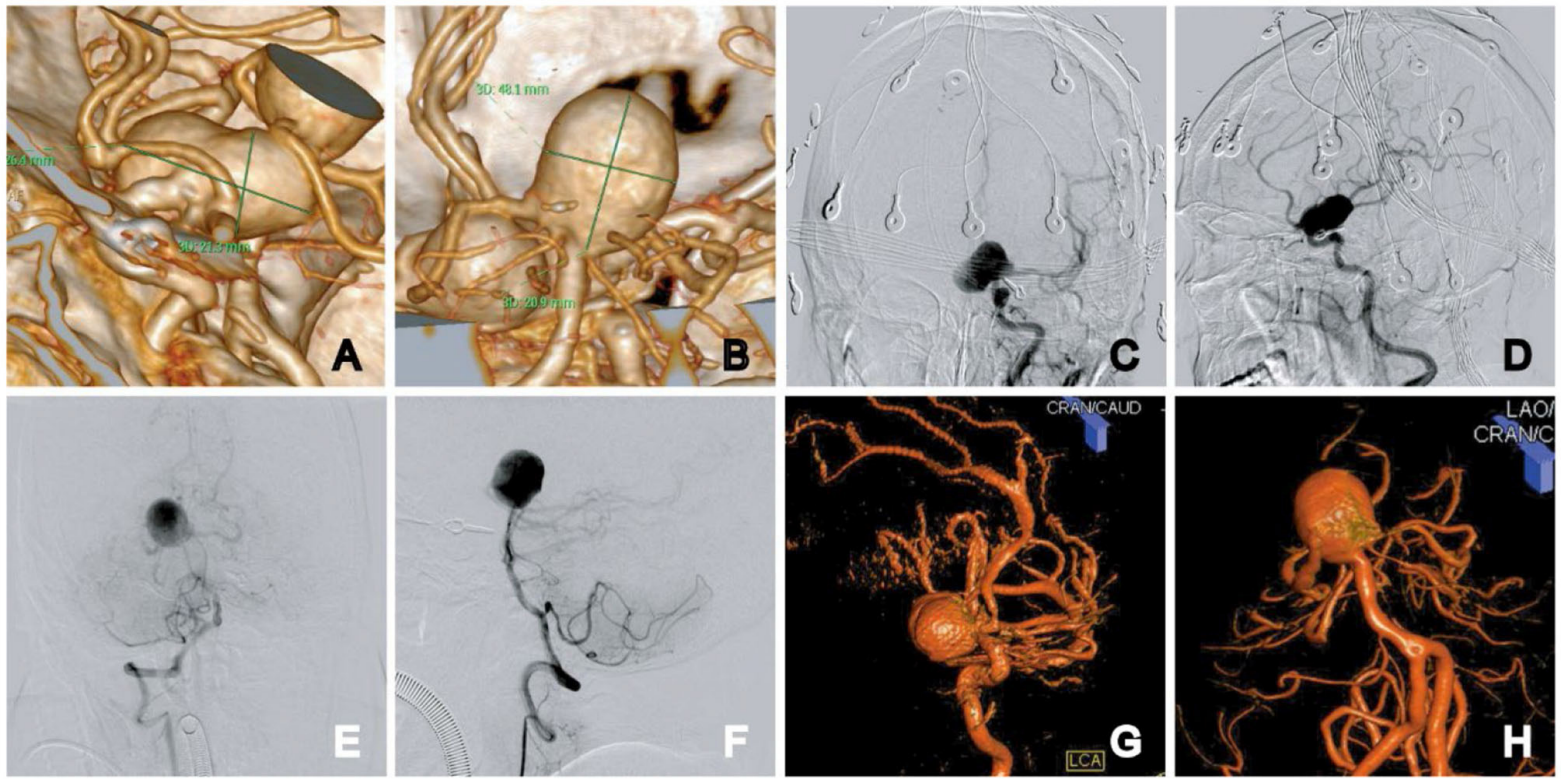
Initial diagnostic workup. CT angiogram images show the left ICA supraclinoid aneurysm and the basilar apex aneurysm: 3D reconstruction measurement, the aneurysm size was 26.4 × 21.3 mm in the left ICA supraclinoid aneurysm (A) and 20.9 × 18.1 mm in the basilar apex aneurysm (B). (C–F) Anteroposterior and lateral views of the left ICA supraclinoid aneurysm (C, D). Anteroposterior and lateral views of bilateral vertebral arteries (E, F). (G, H) 3D rotational angiography of the supraclinoid aneurysm (G) and the basilar apex aneurysm (H).
